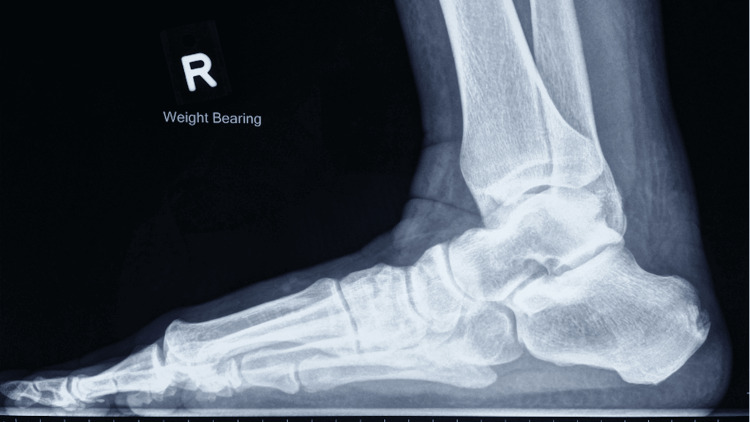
Pre-operative weight-bearing lateral radiographs of the patient’s right foot.
